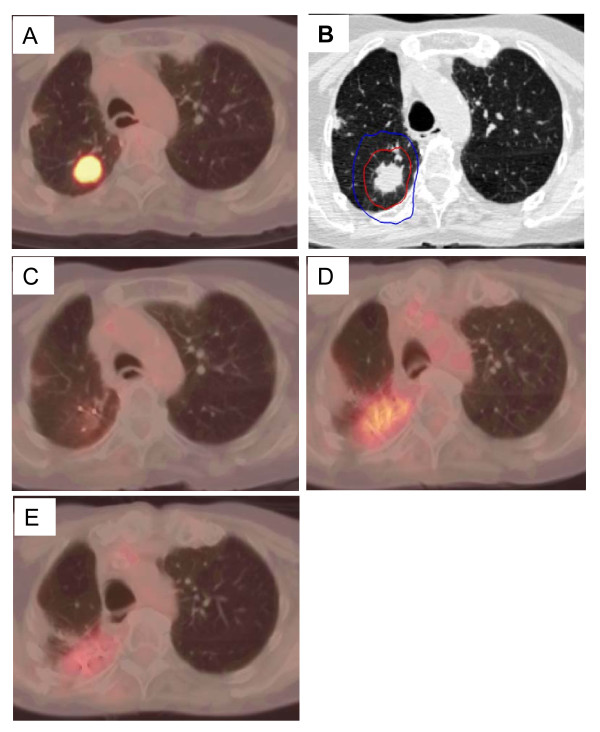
Right upper lobe clinical stage IA NSCLC treatment planning PET/CT with a tumor SUV_max _of 10.5 (A), planned radiation dose distribution (B: the planning treatment volume receiving 45 Gy shown in red and the 30 Gy isodose line in blue), and PET/CT at 6, 12, and 18 months post-treatment (C, D and E) show an initial decrease in tumor SUV_max _to 1.5 followed by a transient radiation induced increase (tumor SUV_max _= 4.0) which resolves by 18 months (tumor SUV_max _= 2.5).
